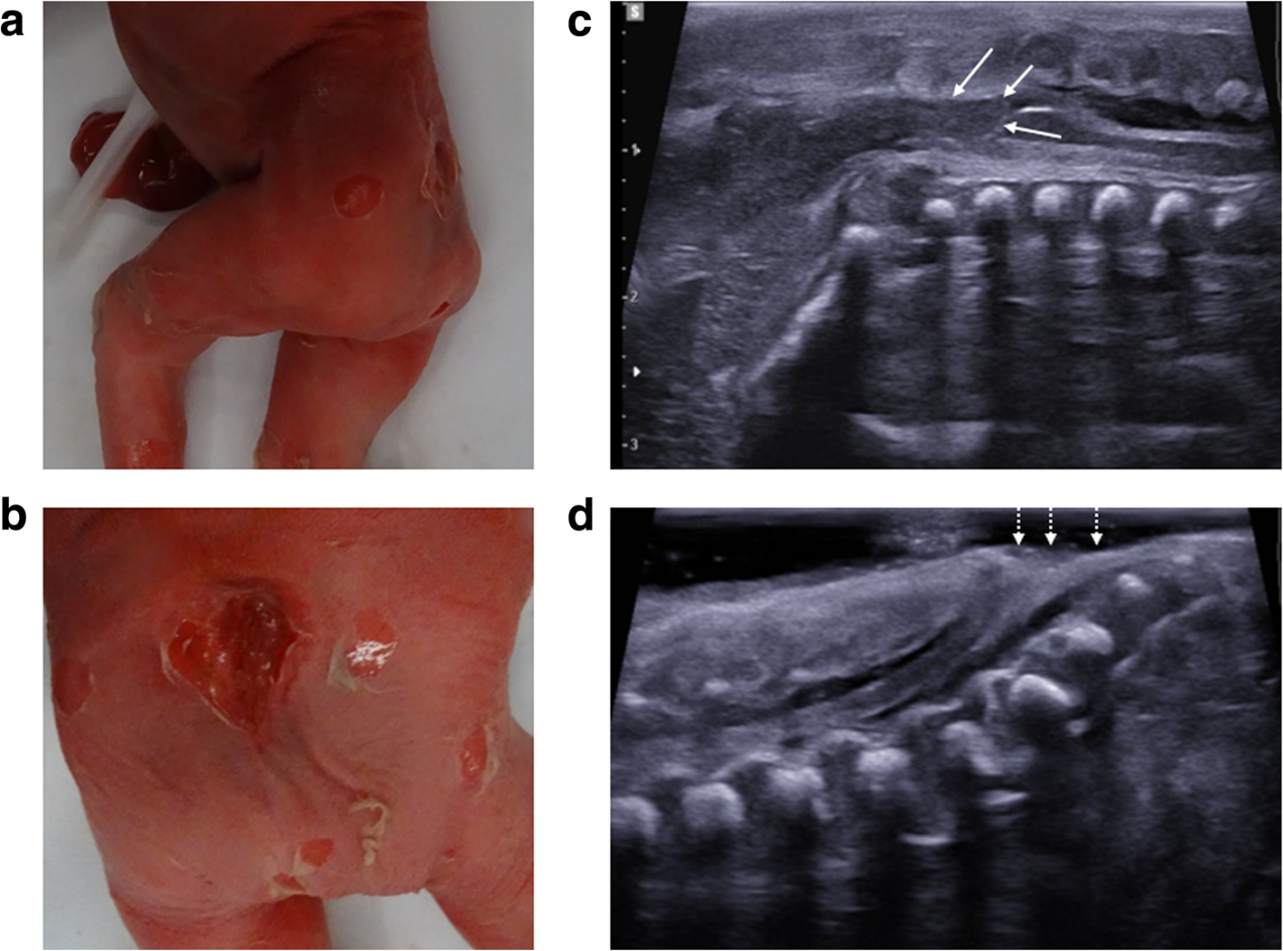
Neural tube defect following termination of pregnancy in a foetus at 25 weeks gestation. A small neural tube defect is identified on external examination at autopsy with the foetus viewed from the left side (a) and from behind (b). On the corresponding post-mortem ultrasound performed 4 days after death, the sagittal view of the cranio-cervical junction (c) demonstrates herniation of the cerebellar tonsils (solid white arrows) into the upper cervical spinal canal. The sagittal ultrasound image of the lumbosacral spine (d) demonstrates the small neural tube defect (dashed arrows)
